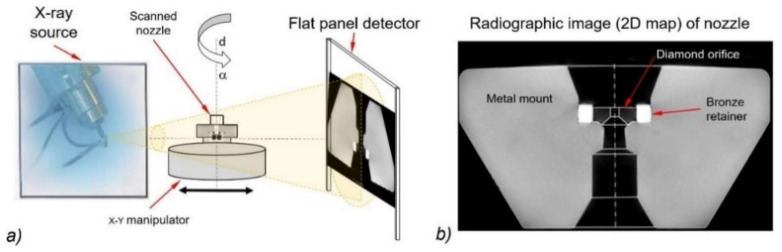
X-ray CT scheme: (a) projection of the water nozzle using the CT method; (b) 2D image of the water nozzle.
